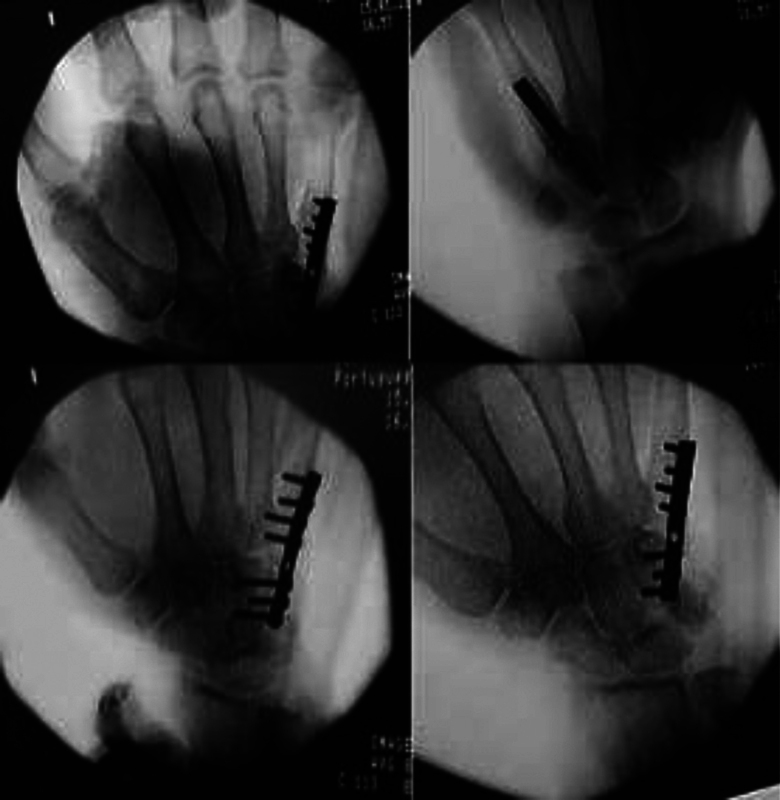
Case 2: anteroposterior, posteroanterior, and oblique radiographs in the immediate postoperative period of tumor resection and reconstruction with Free osteoarticular metatarsus autograft.
